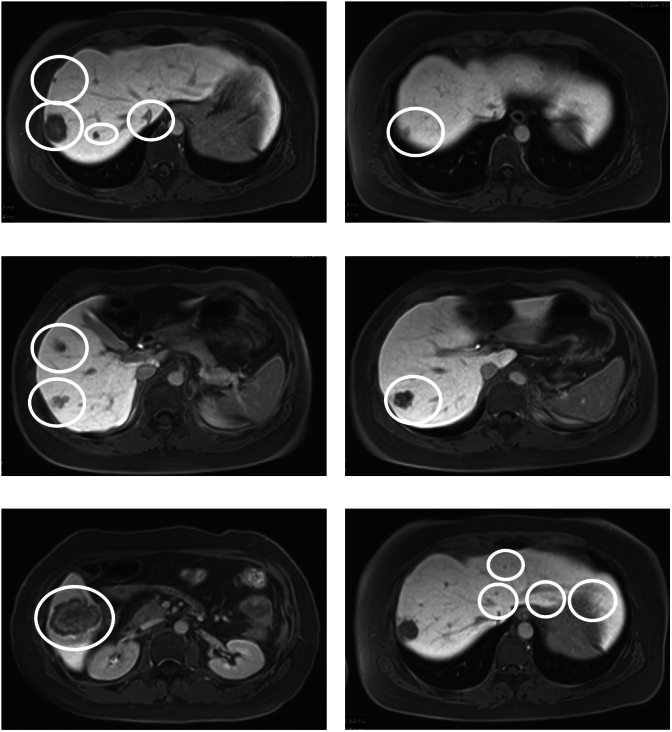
Ethoxybenzyl-magnetic resonance imaging (EOB-MRI) detected 13 masses with a maximum diameter of 37 mm in the liver (white circles).
